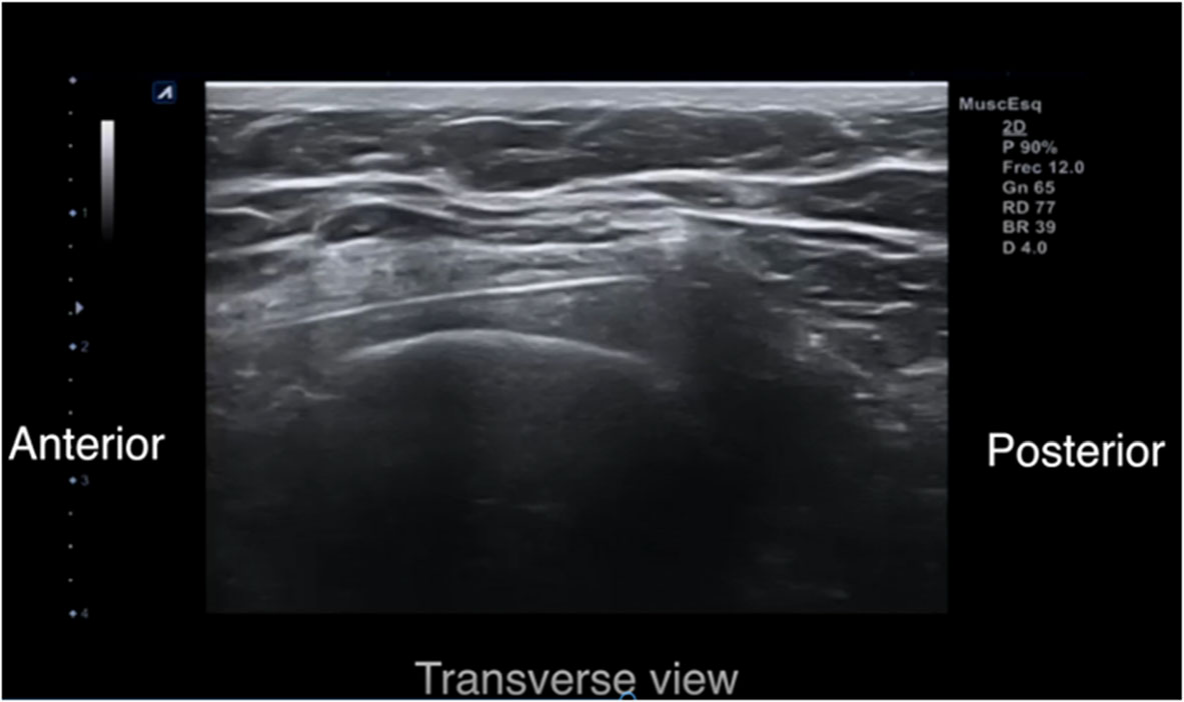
The hook-knife reaches the posterior limit of the proximal ITB. The fibers of the muscle are clearly different from the ITB. Ultrasound image
